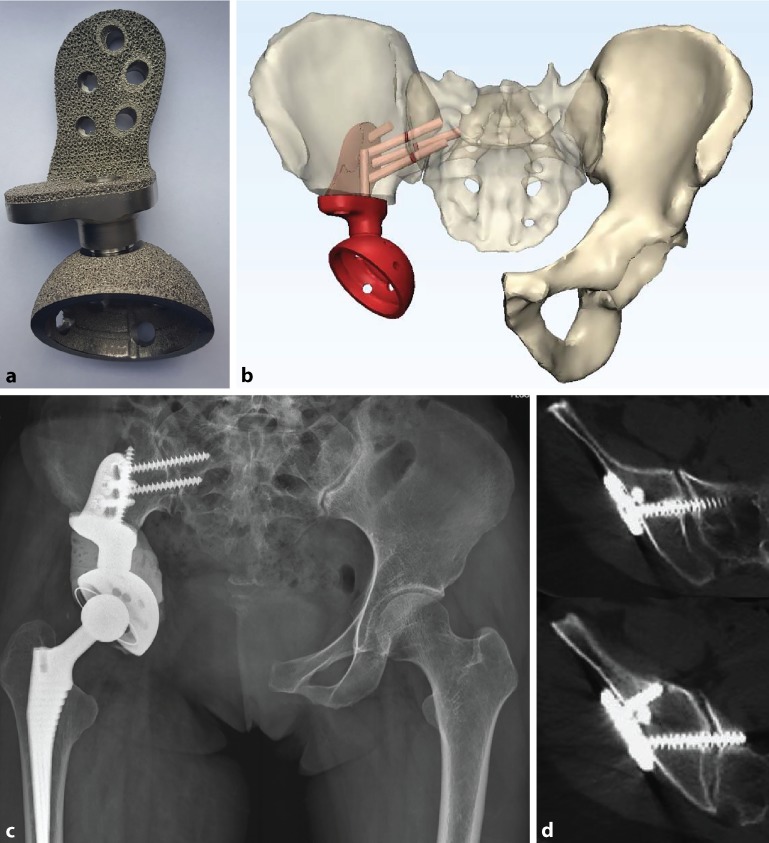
A 39-year-old patient with right acetabulum chondrosarcoma resection, reconstructed with an electron-beam melting three-dimensionally printed porous modular hemi-pelvic endoprosthesis (a) with preoperative virtual planning (b). Radiograph at 2 years (c), with computed tomography showing osteointegration (d)
